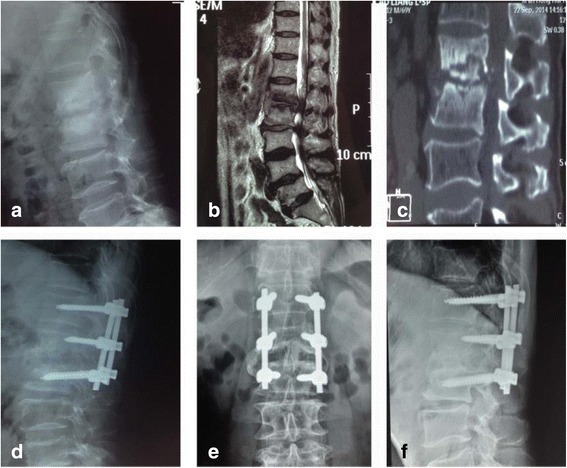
Images from a 70-year-old man with lumbar spinal tuberculosis who underwent one-stage posterior debridement, bone grafting, and instrumentation with local continuous chemotherapy and postural drainage. a–c Preoperative X-ray, MR, and CT images show the destruction of L1 and L2 and paravertebral abscess formation. d Postoperative lateral X-ray showing fixation of T12–L2. e, f X-rays obtained 6 months postoperatively show successful interbody bony fusion
